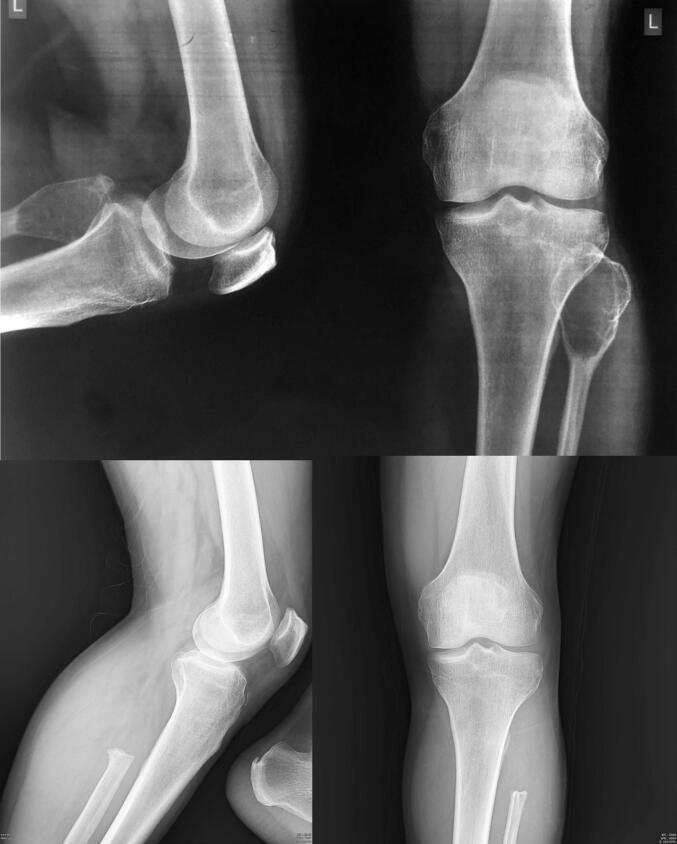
Plain radiographs of the knee in a young male presenting with pain and a palpable lateral proximal leg mass. Images demonstrate a lytic, expansile lesion involving the proximal fibula with cortical thinning and expansion, consistent with a diagnosis of giant cell tumor (GCT), subsequently managed by surgical resection.
